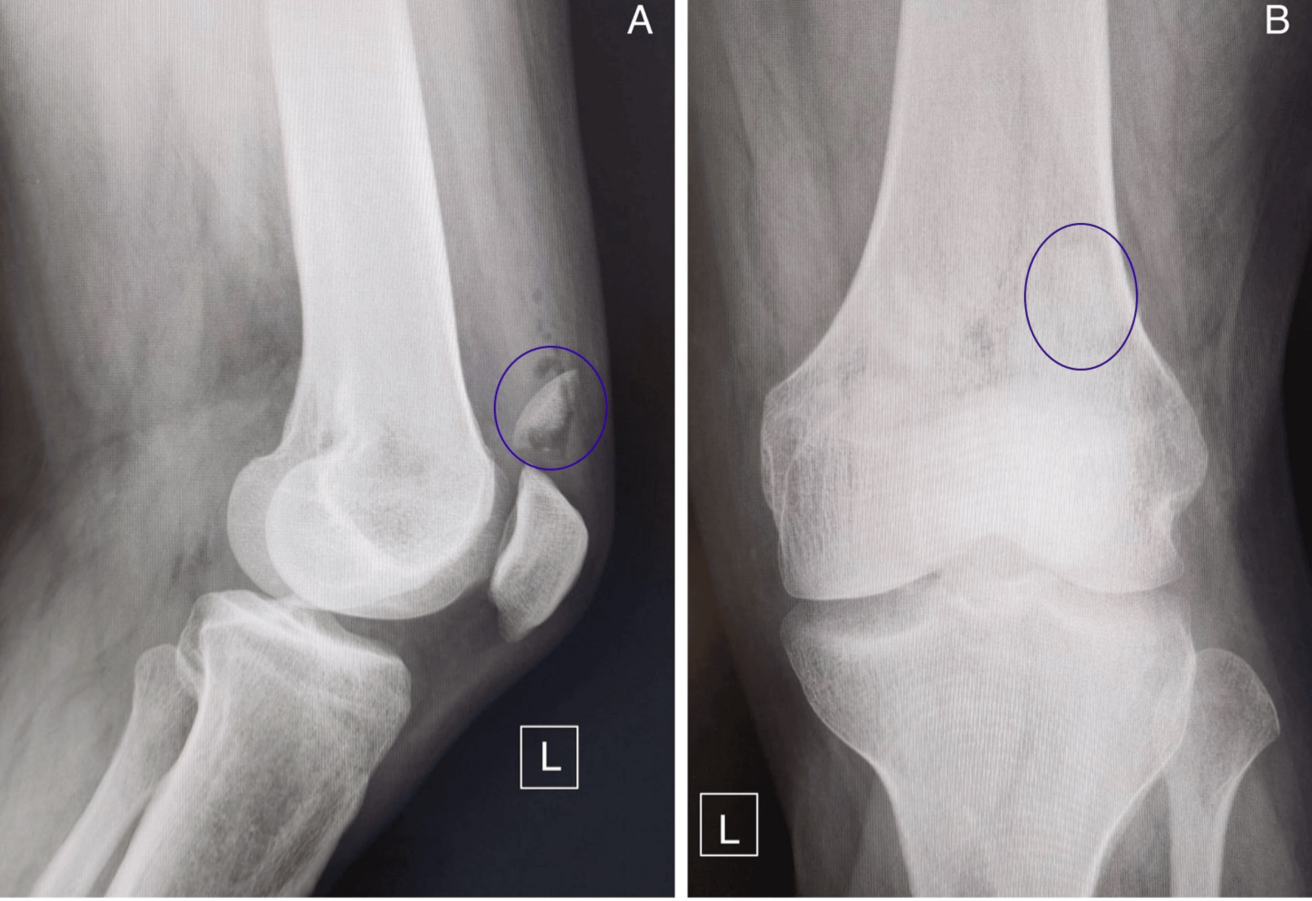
Left knee X-rays - (A) lateral and (B) anteroposterior views - obtained at initial presentation, with the osteochondral fragment highlighted by a blue circle.
